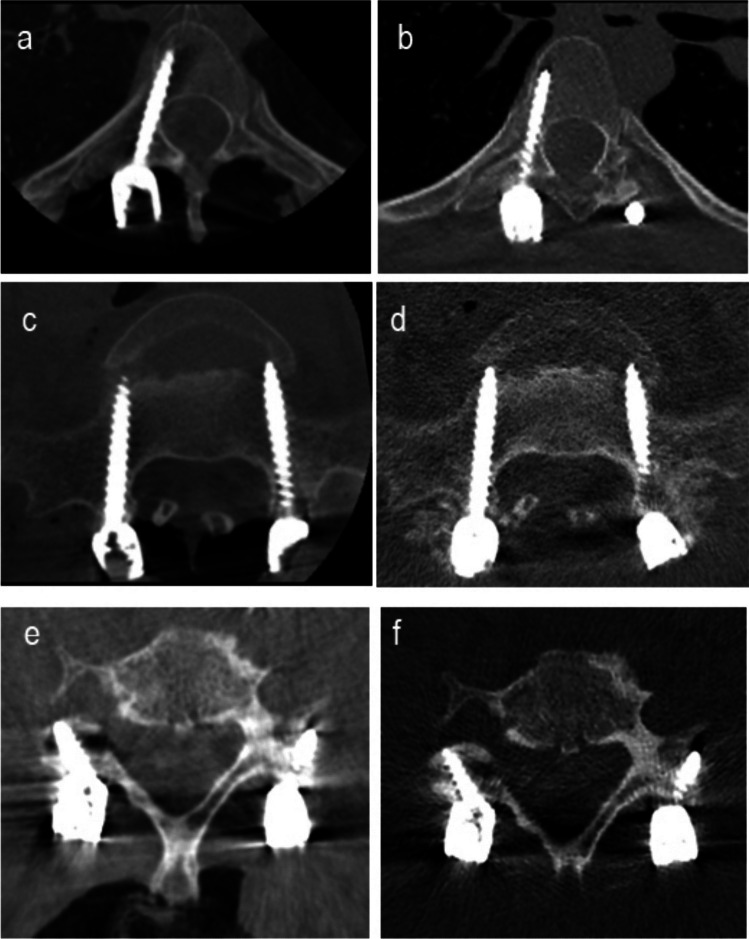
Example of corresponding images of cone-beam computed tomography (CBCT) and multidetector computed tomography (MDCT) images in the axial plane for assessment of image quality in intra- and postoperative spine surgery. Images depict CBCT (a, c, e) and MDCT (b, d, f). Thoracic vertebrae with pedicle screws (a, b). Lumbar vertebrae with pedicle screws (c, d). Cervical vertebrae with lateral mass screws (e, f)
